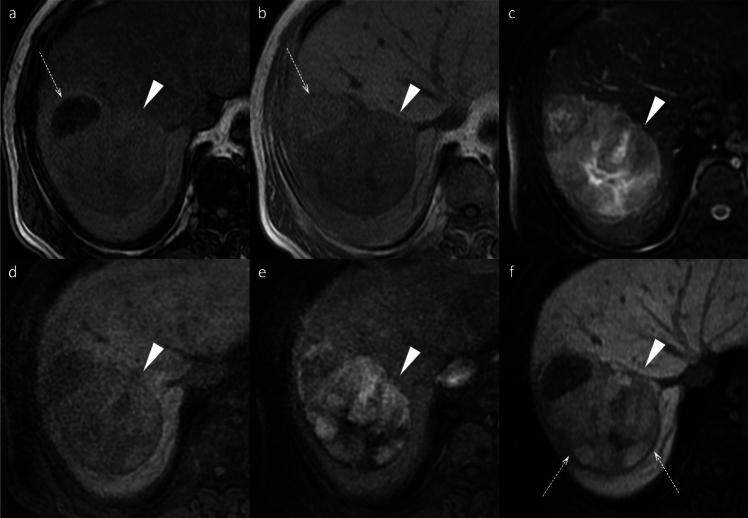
Hepatocellular carcinoma (HCC) showing inhomogeneous hyperintense in the hepatobiliary phase in a 76-year-old man. HCC (arrowheads) includes an area decreasing in signal intensity on the opposed-phase image (a) versus the in-phase image (b), confirming the presence of intralesional fat (arrows). HCC (arrowheads) also shows slight hyperintensity on the fat-suppressed T2-weighted image (c), inhomogeneous hyperintensity on the pre-contrast image (d), and non-rim arterial phase hyperenhancement (e). In the hepatobiliary phase, HCC (arrowheads) displays inhomogeneous hyperintensity (arrows) (f)
